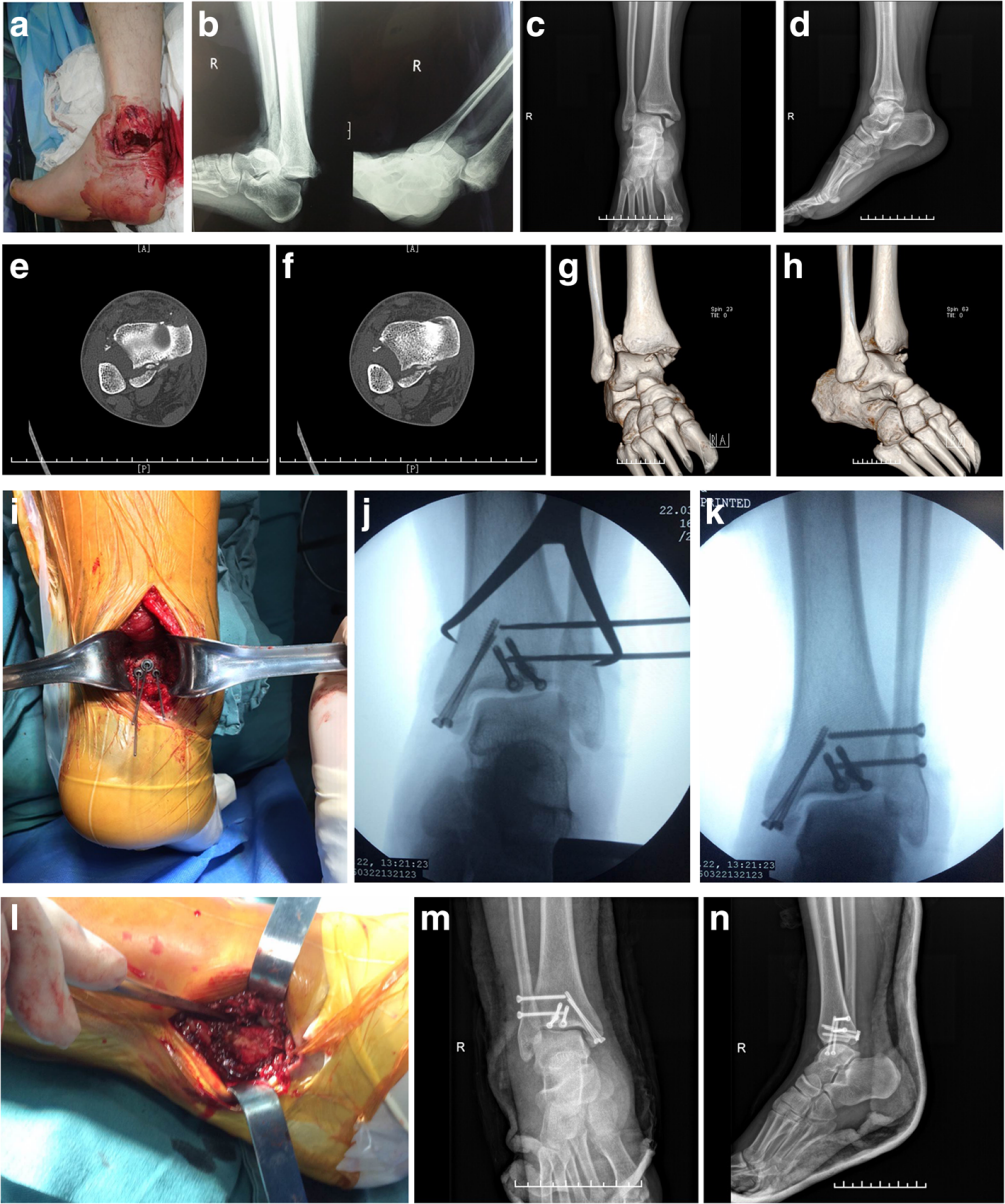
Prereduction (a, b) and postreduction (c anteroposterior; d lateral) radiographs from a 44-year-old man after traffic accident (typical Logsplitter injury). The CT plain scan (e, f) with three-dimensional reconstruction (g, h) revealed increased tibiofibular width and Volkmann fragments. During the surgery, Volkmann fragments were fixed using the cannulated screws (i); syndesmotic screw fixation was performed by screwing 3.5-mm syndesmotic screws (j. k); medial malleolus fragments were fixed using the cannulated screws (l). The anteroposterior (m) and lateral (n) radiographs at the final follow-up showed satisfactory reduction
